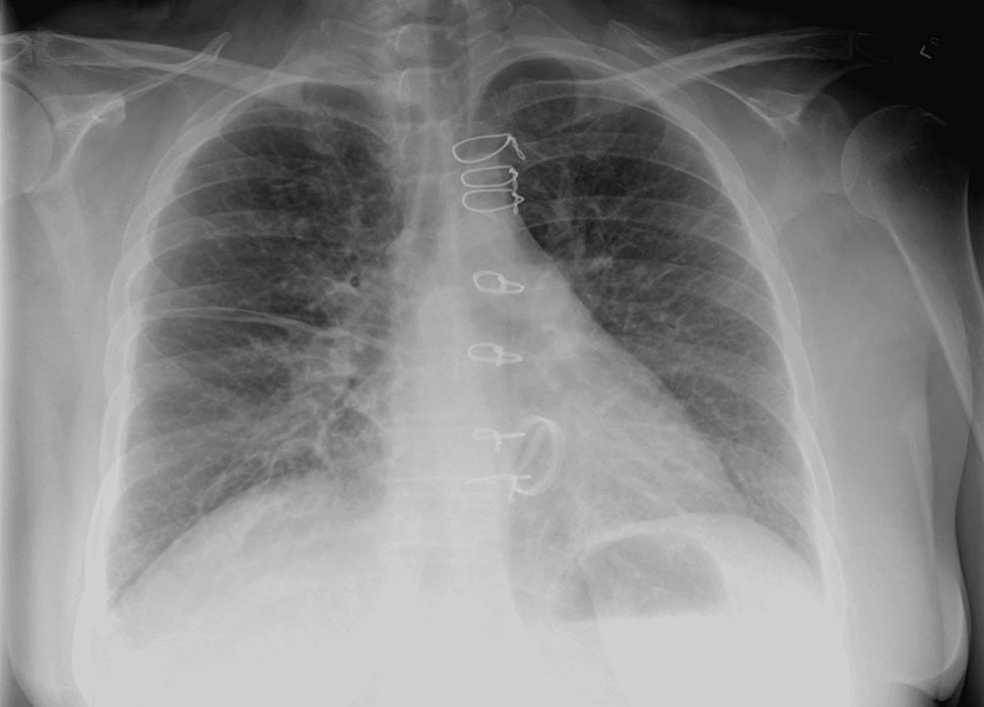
Chest X-ray showing normal cardiac shadow with a mechanical mitral valve in place and a prominent pulmonary vascular marking with bilateral diffuse interstitial edema.
